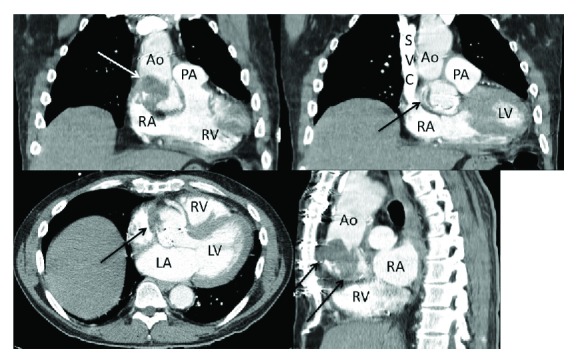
Axial, sagittal, and coronal contrast-enhanced CT images of the chest showing a fungal ball in the aortic root adherent to the prosthetic valve (arrows). Ao = aorta; PA = main pulmonary artery; RA = right atrium; LA = left atrium; RV = right ventricle; LV = left ventricle; SVC = superior vena cava.
